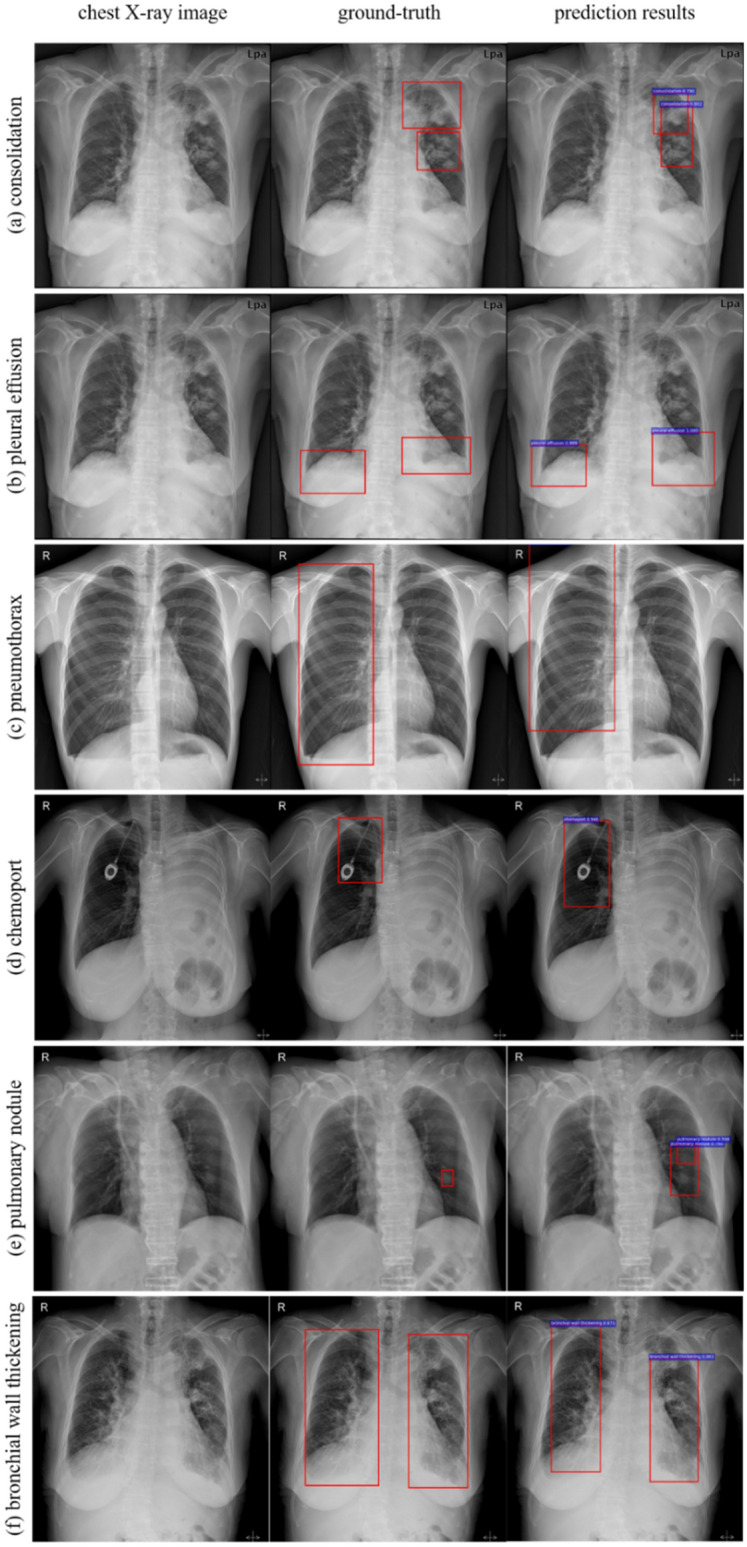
Examples of prediction results of multi-object detection in this study. Examples of the predicted results are shown in Figure 4. Overall, twelve classes are described in this model, such as pleural effusion, atelectasis, pulmonary nodule, cardiomegaly, consolidation, emphysema, pneumothorax, chemo-port, bronchial wall thickening, reticular opacity, pleural thickening, and bronchiectasis. From left to right, actual chest X-ray image, ground-truth (expert-described data labels), and results from prediction model are shown.
